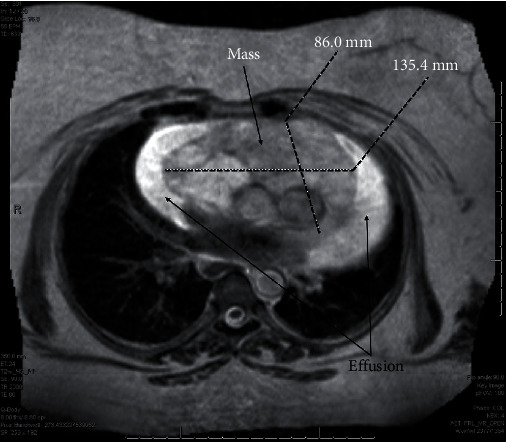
Magnetic resonance imaging at our institution revealed a lesion measuring 8.6 × 13.5 cm.
